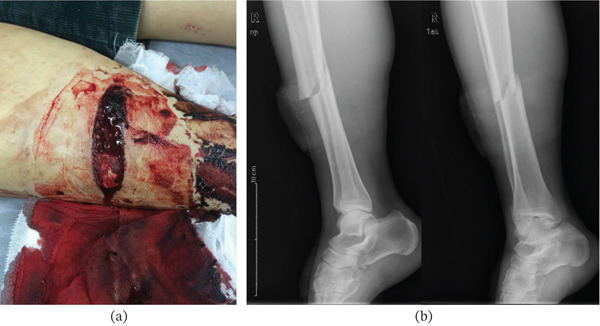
(a) An adult patient sustained an open tibial fracture by a chainsaw splash. (b) Preoperative radiographs: lateral (left) and oblique (right) views showing a simple oblique fracture of the tibial shaft (AO/OTA 42A2). The oblique view was obtained due to patient positioning constraints.
